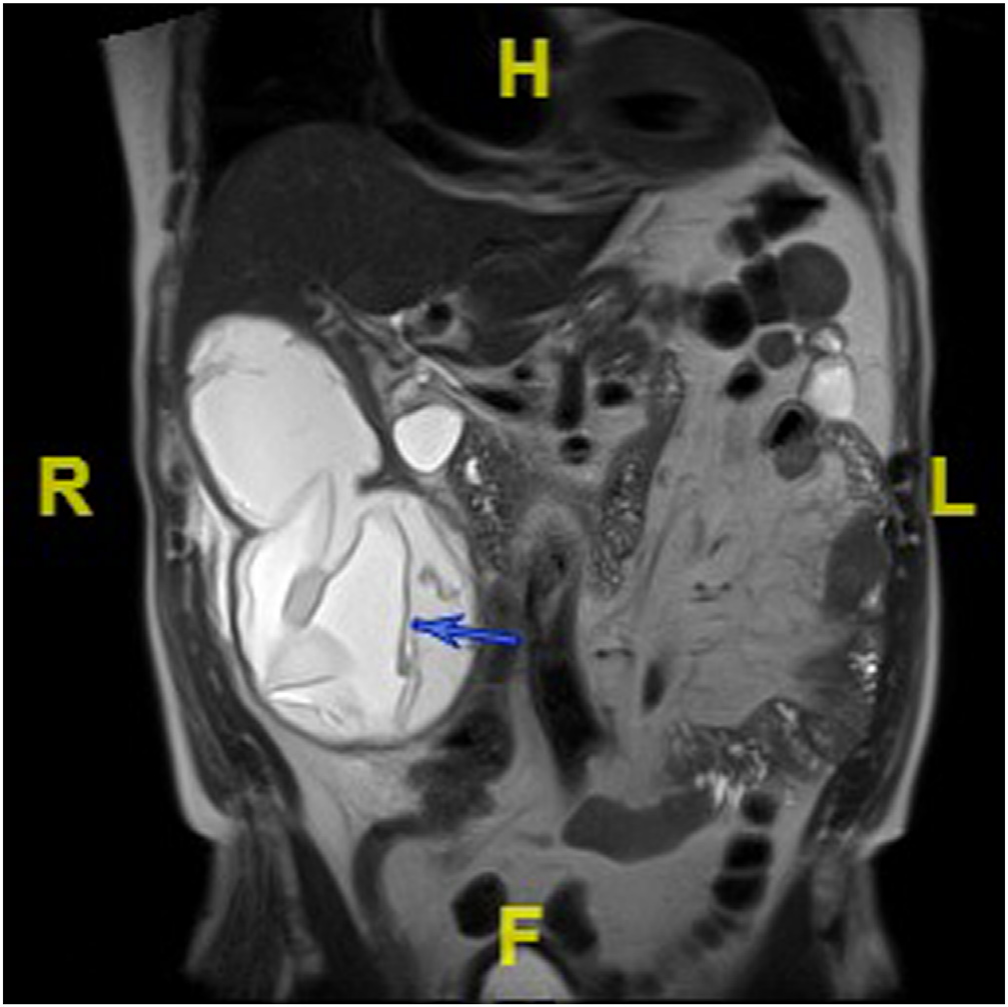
Magnetic resonance imaging. Coronal section in T2-weighted pulse sequence. Cystic bilobate lesion with exophytic part. WHO Classification: CE3a: Liquid cyst with detached membrane appearance.
